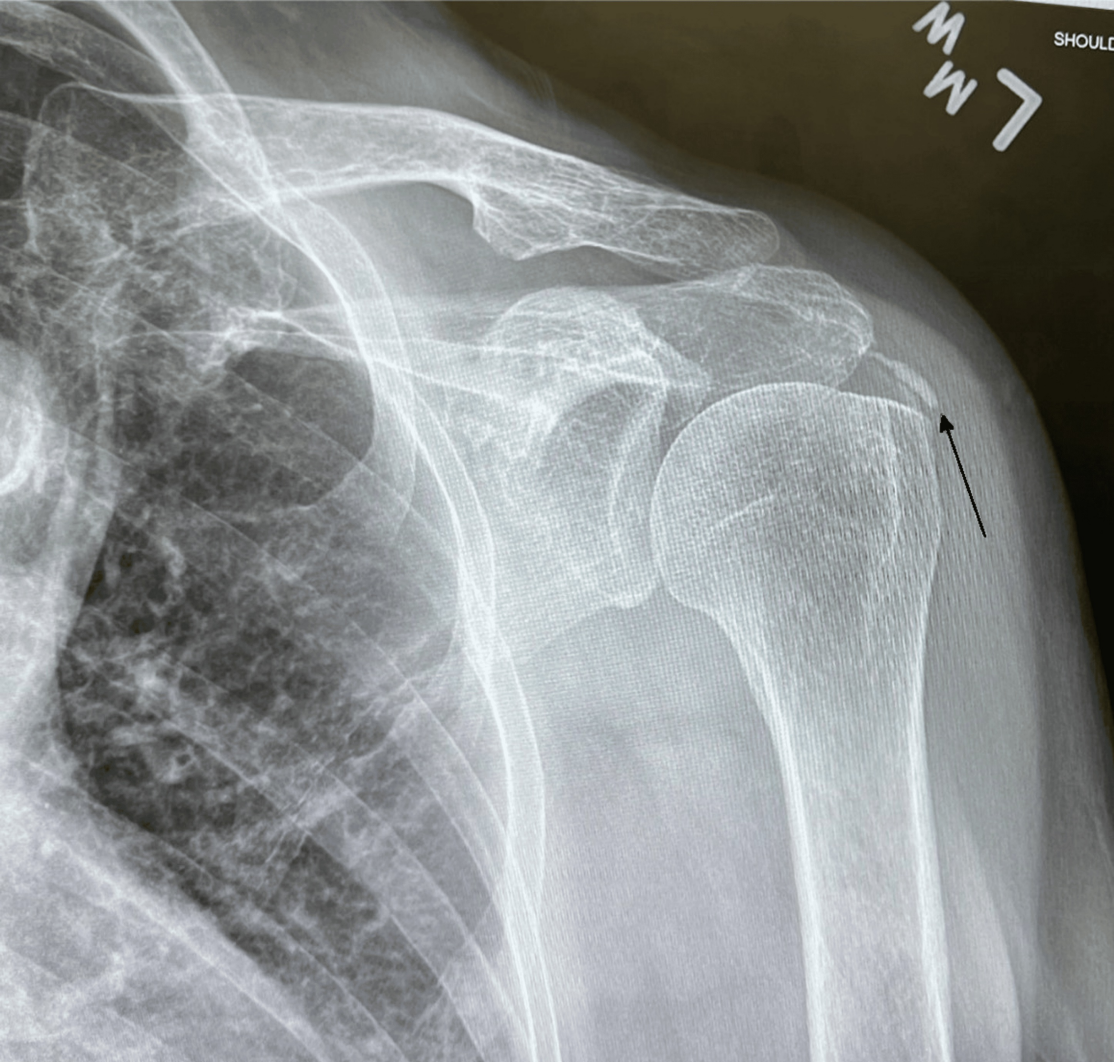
X-ray image of left shoulder showing a calcific deposit, indicated by the arrow.
